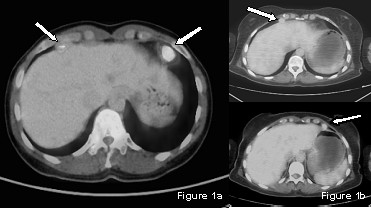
PET-CT appearance of bilateral cardiophrenic angle node metastasis in the two cases reported.
